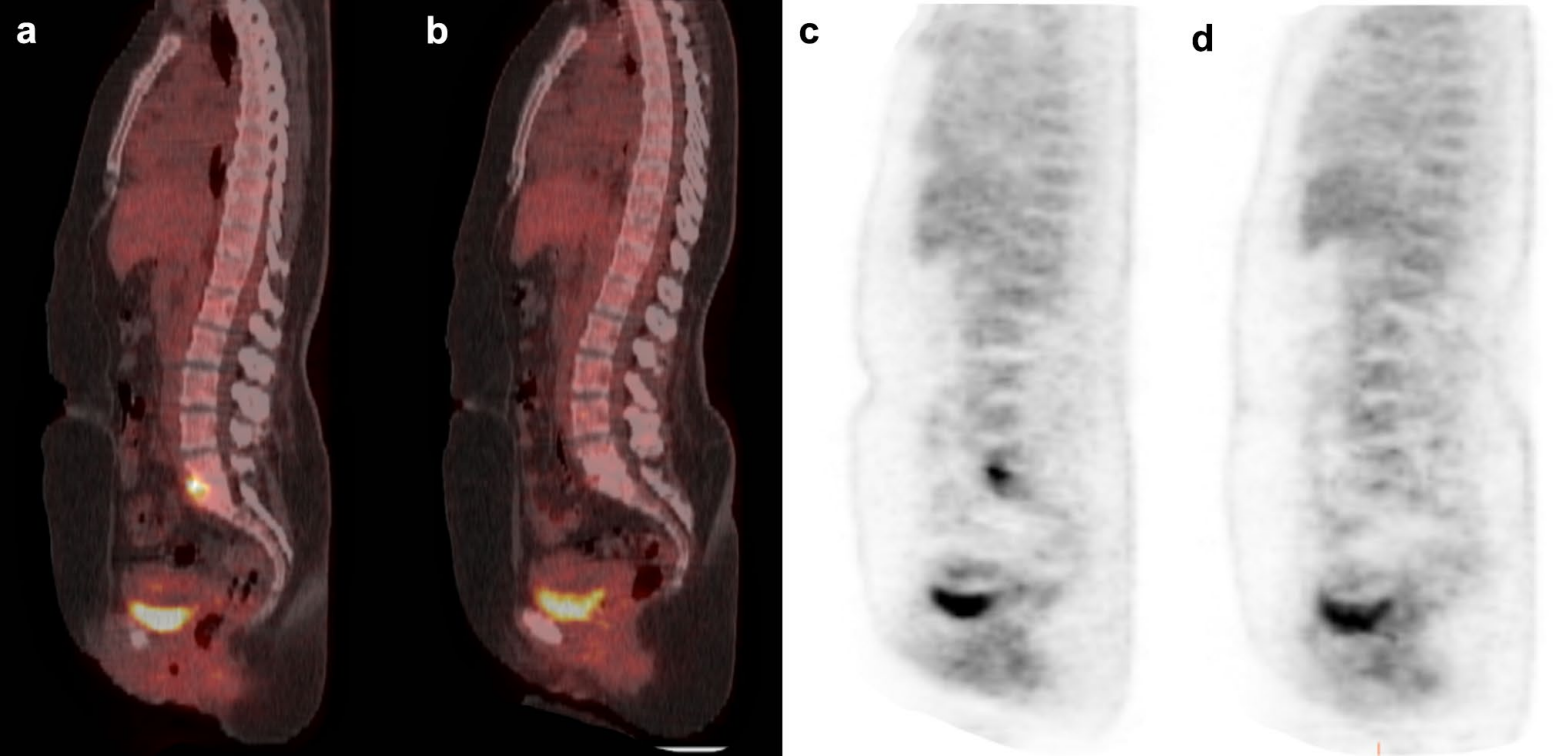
^18^F-FDG-PET/CT images before and after therapy in a 35-year-old woman with Candidemia (*Candida albicans*). ^18^F-FDG-PET/CT showed increased ^18^F-FDG-accumulation at the level L5–S1 (a, c), spondylodiscitis was confirmed by spinal biopsy which also showed *Candida albicans*. The ^18^F-FDG-PET/CT was repeated after 5 months of therapy with fluconazole and showed only minimal ^18^F-FDG-uptake (b, d) which was interpreted as reactive changes after treatment for spondylodiscitis
